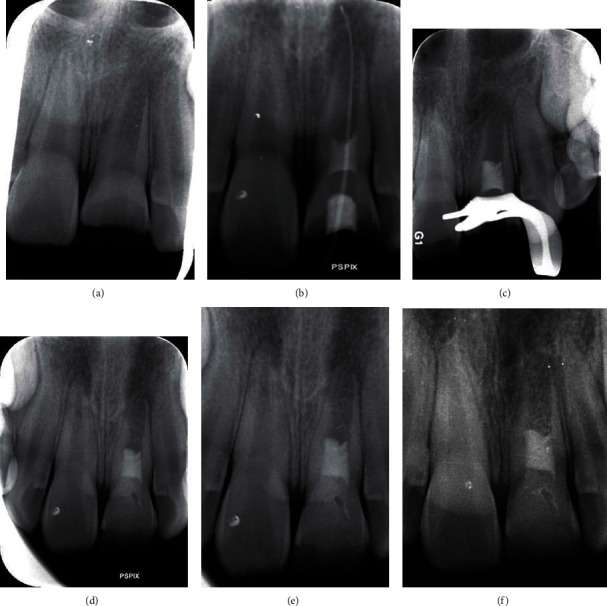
The radiographic images showing (a) the initial preoperative condition, (b) tracing the mucosal sinus tract with a gutta percha point, (c) second try, (d) 3-month recall, (e) 9-month recall, and (f) 18-month recall.
